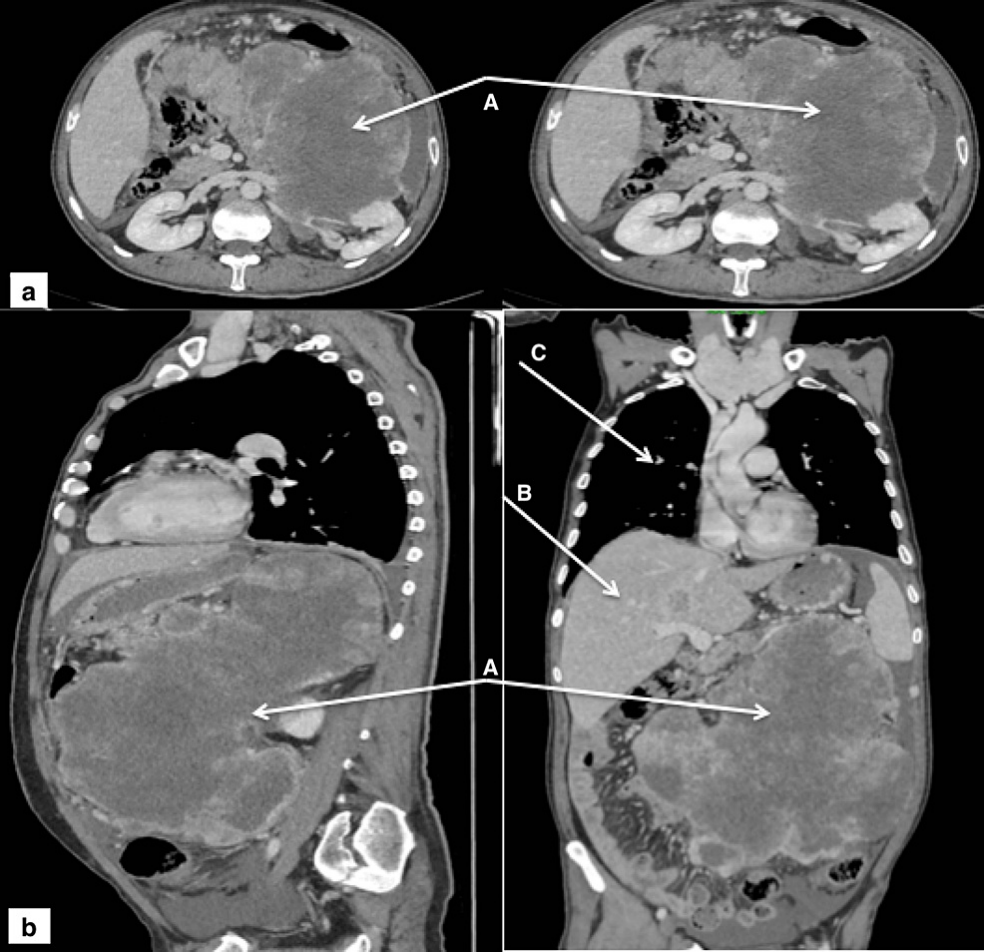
Thoracoabdominal CT scan: (a) cross-section and (b) coronal section. A: Left adrenal mass measured 13 x 16 x 24 cm, massively necrotic. B: Secondary hepatic localization. C: Secondary pulmonary localization.
